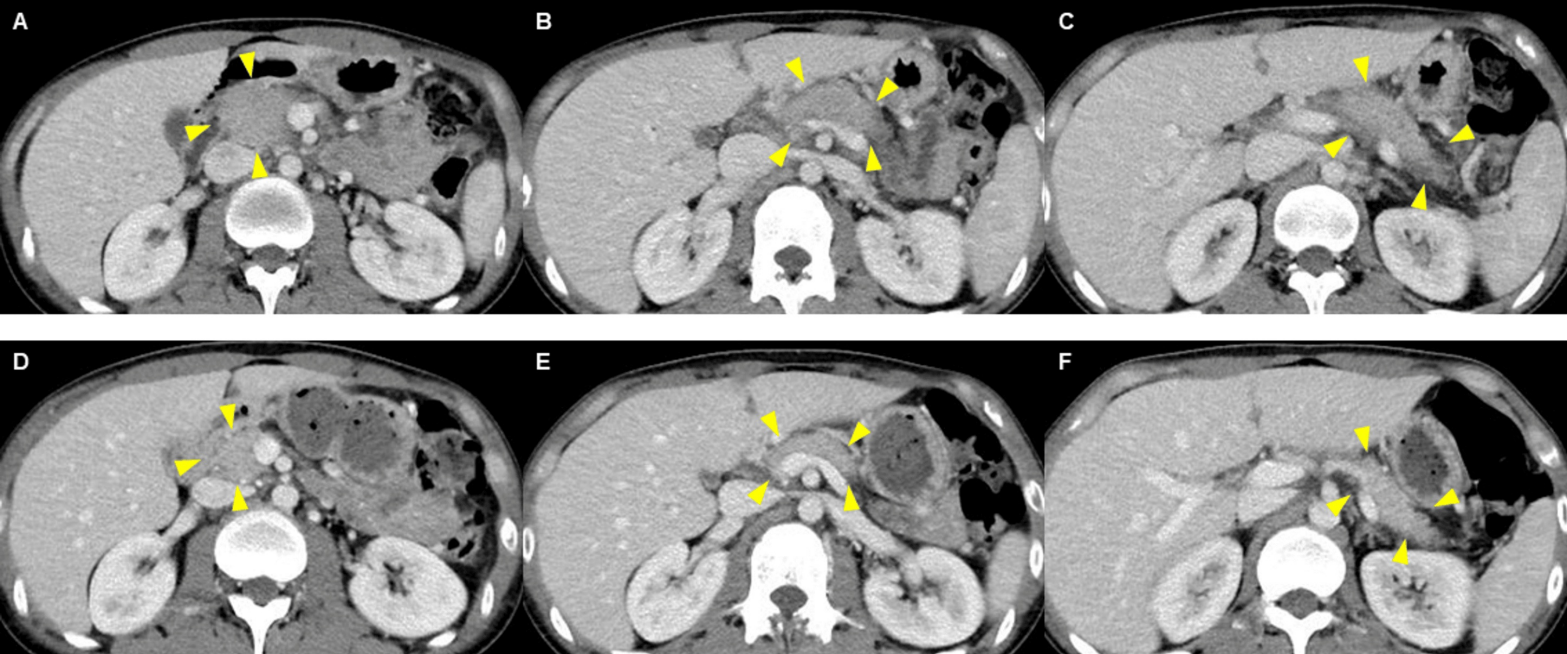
Computed tomography (CT) findings. A diffusely enlarged pancreas with increased density of the surrounding fatty tissue is shown before treatment (A-C) (arrows), with notable improvement shown in pancreatic appearance two weeks after steroid therapy (D-F) (arrows).
